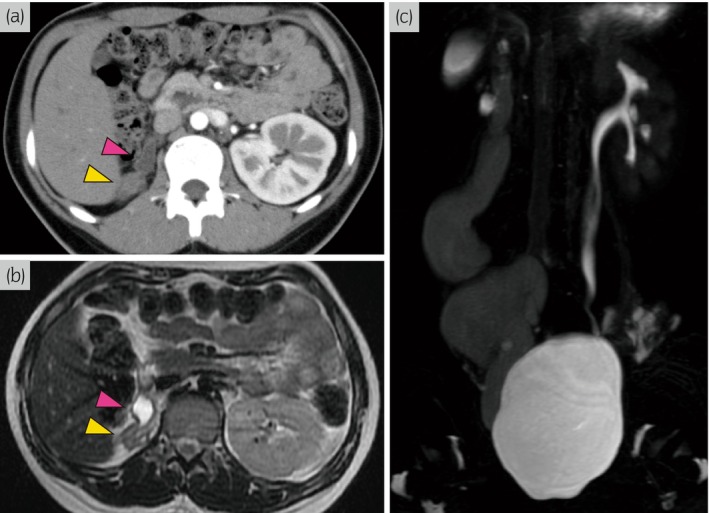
Imaging findings. (a) Enhanced CT image shows the right atrophic kidney (yellow arrow) and hydroureter (pink arrow). (b) MRI shows right atrophic kidney (yellow arrow) and hydroureter (pink arrow). (c) MRU reveals marked hydroureter on the right side.
